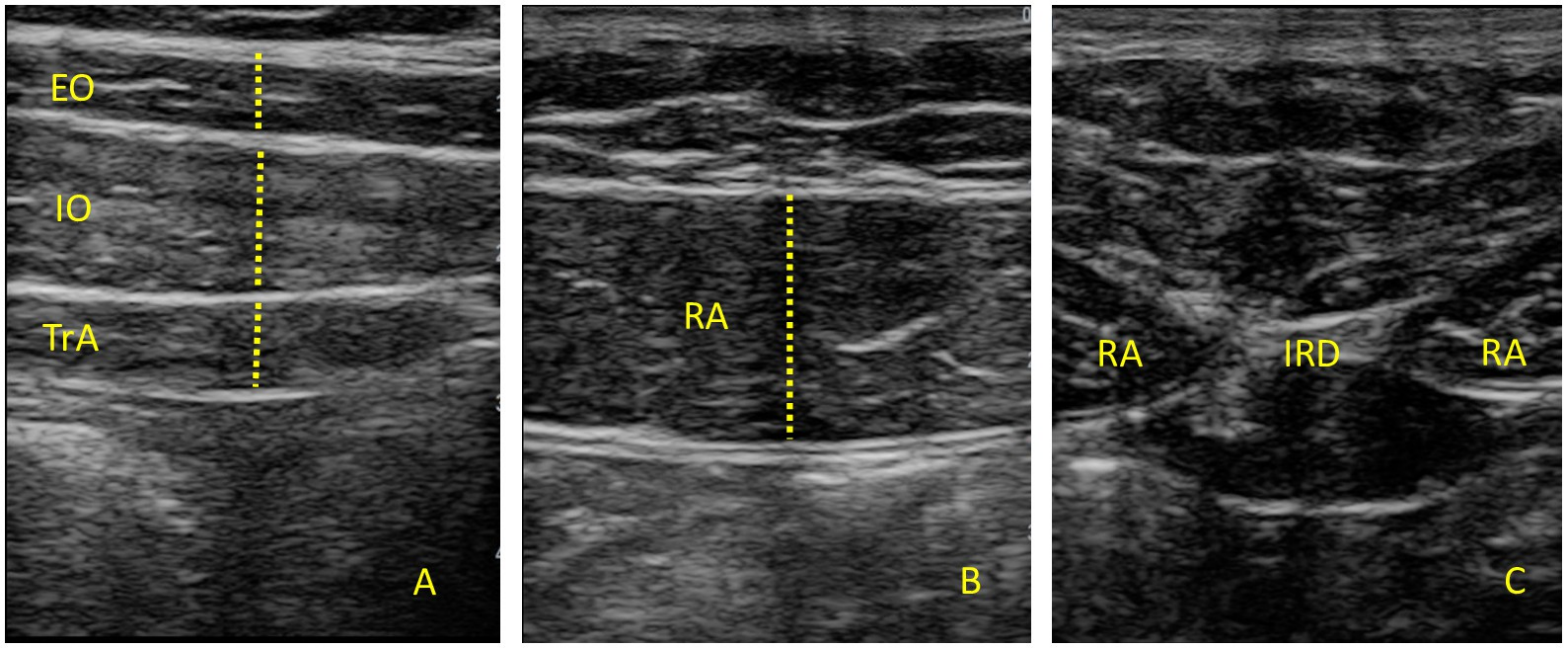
Ultrasonography of the abdominal wall muscles and IRD.
